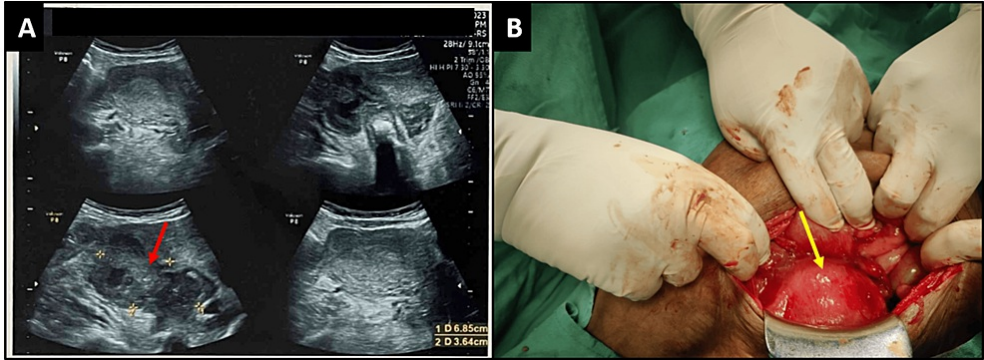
Ultrasound of the right adnexa shows a heteroechoic lesion of size 68 mm x 36 mm that was not separately seen from the right ovary (A). The intraoperative image reveals a bulky uterus with an edematous fallopian tube (B).
